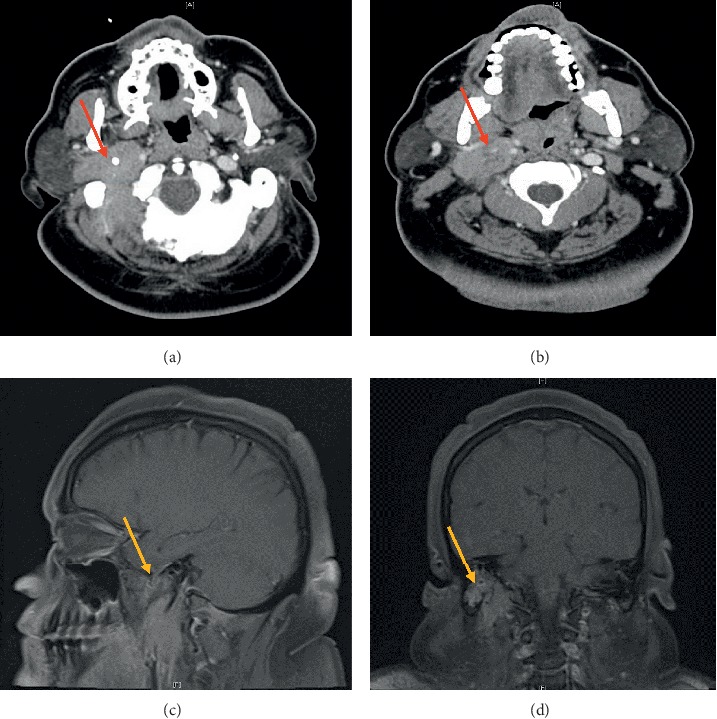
CT scan of the neck and soft tissue showing and infiltrative mass on the right skull base extending to the right jugular and stylomastoid foramen (a) encasing and occluding the right jugular vein (b). MRI confirms a soft-tissue mass on the skull base without intracranial involvement (c and d).
